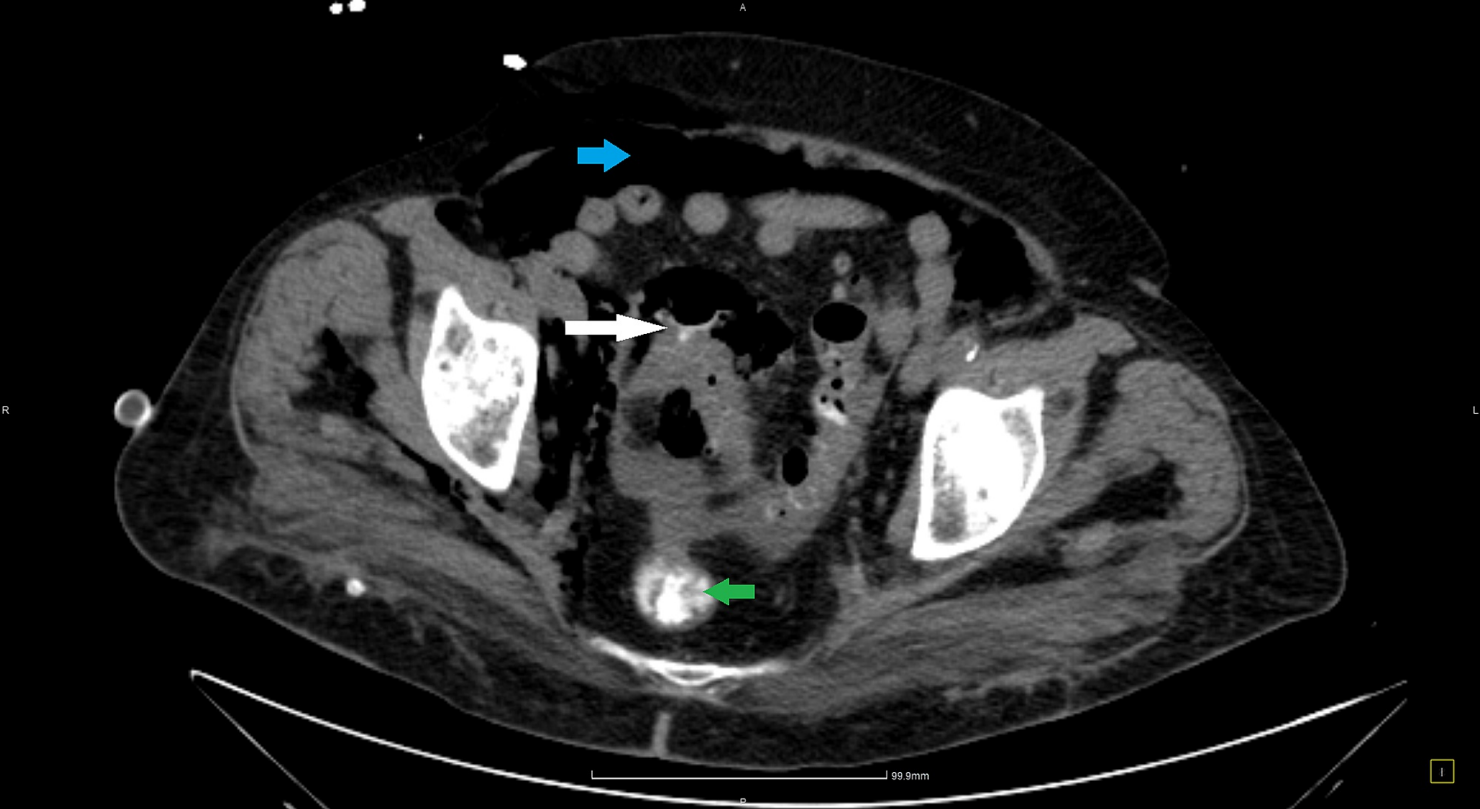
CT with rectal contrast. Green arrow: contrast in rectum; white arrow: extraluminal contrast; blue arrow: pneumoperitoneum. CT, computed tomography
